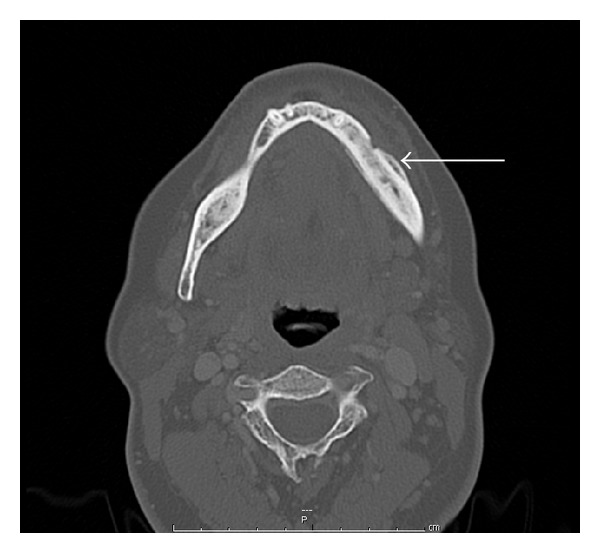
CT scan of the mandible. The CT scan demonstrates thickening of the mandibular body (arrow) with adjacent tissue stranding. This was thought to represent metastatic disease.
